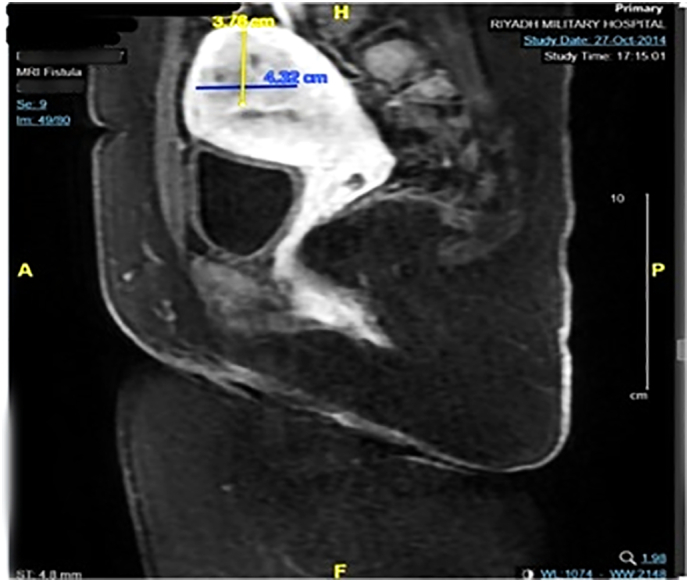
Pelvic MRI showing a uterine fibroid.
